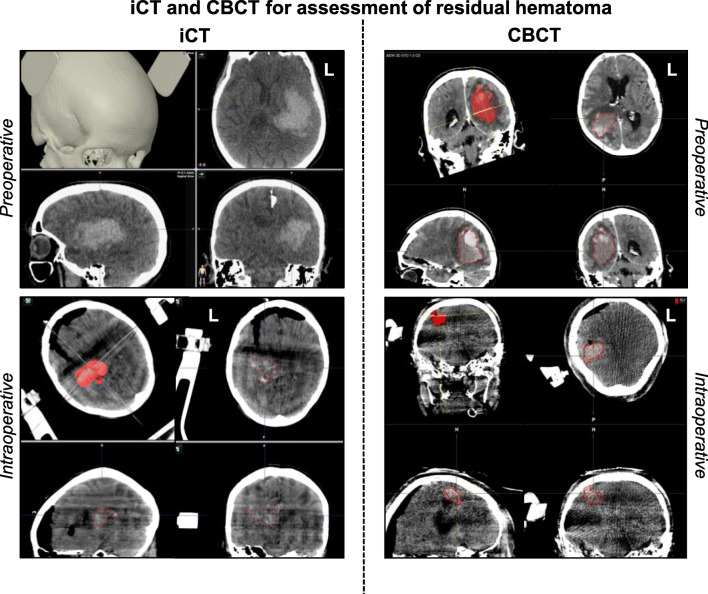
Intraoperative control imaging for hematoma detection. The preoperative CT images of patients 2 and 14 (upper panels) are displayed in comparison to intraoperative iCT and CBCT scans (lower panels) with residual hematoma for evaluation of intraoperative hematoma assessability after evacuation. L, left
